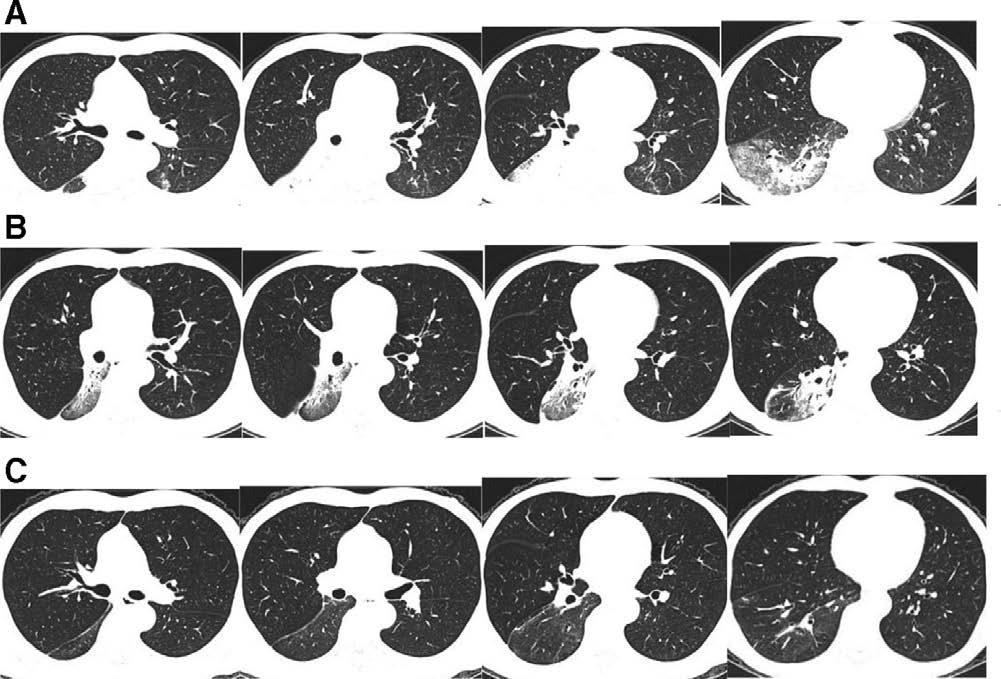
Computed tomography. A: There was a large consolidation in the dorsal segment of the right lower lobe, patchy exudation with partial consolidation in the basal segment of the right lower lobe, and right lower hilar lymphadenopathy (December 7, 2020); B: The lesion was obviously absorbed (January 4, 2021); C: The lesion was almost completely absorbed with few fibrous cords remaining (February 15, 2021).
